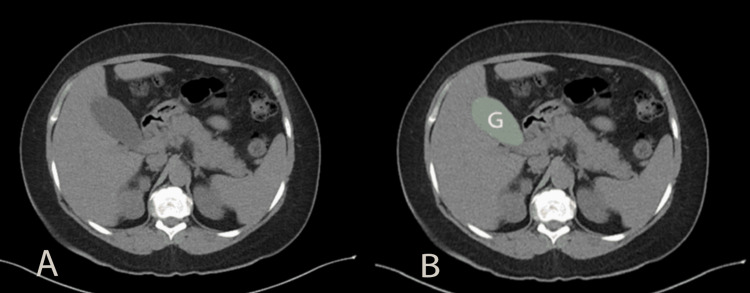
CT abdomen showing gallbladder distention A: Unlabeled axial view demonstrating the gallbladder in situ; B: Axial view with the gallbladder highlighted in green (labeled 'G') for clarity
